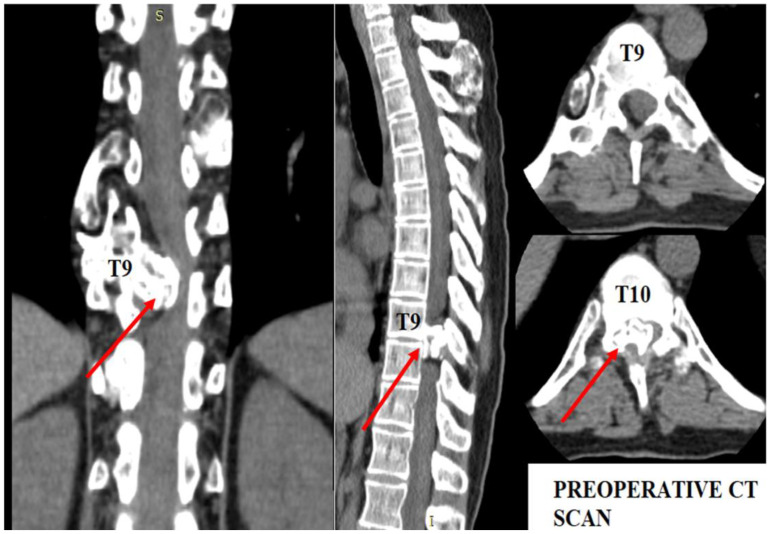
Preoperative CT scan. Note the intracanalar localization determined by the osteochondroma.
